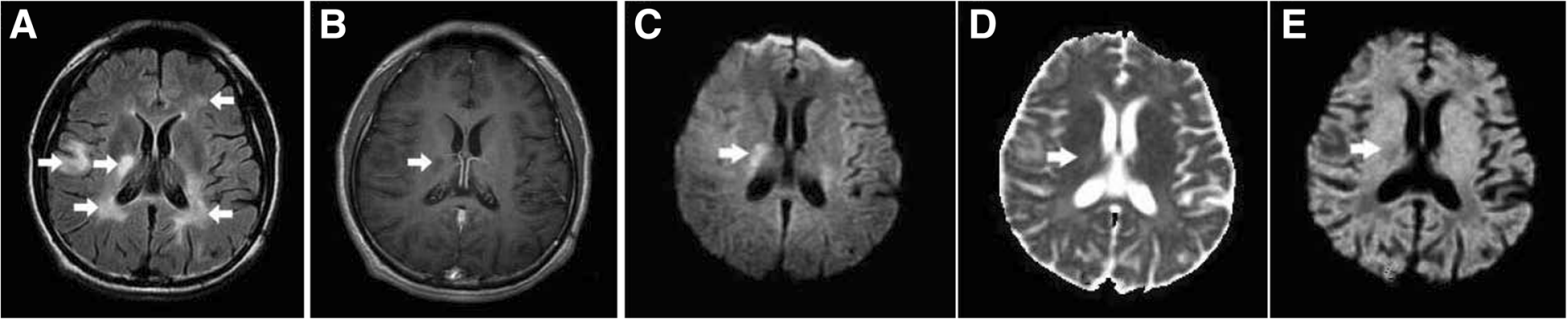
A type IIIB lesion in a patient with MS. (A) Axial T2-FLAIR brain MRI shows multiple demyelinating lesions (arrows) in the bilateral periventricular white matter and one in the right subcortical white matter. (B) All of the lesions show no enhancement on the CE T1WI, including one lesion involving the right periventricular white matter (arrow). (C–E) The lesion (arrow) is hyperintense on the DWI, hyperintense on the ADC map, and hypointense on the eADC map, indicating residual vasogenic edema and the T2 shine-through effect. The hyperintensity on the DWI disappeared on follow-up MRI three months later (not shown).
